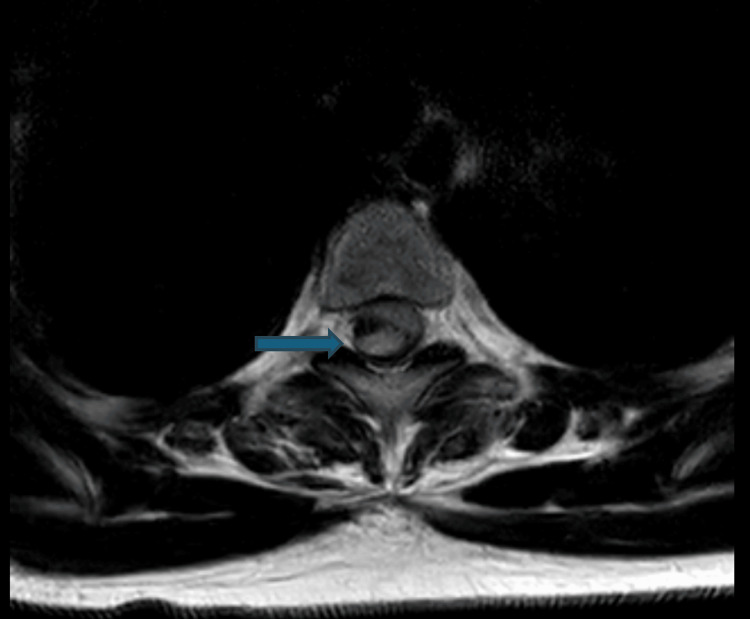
Axial T2-weighted MRI at level T3-T4 showing intrathecal haematoma. The blue arrow indicates the location of the intrathecal spinal haematoma on axial MRI.
